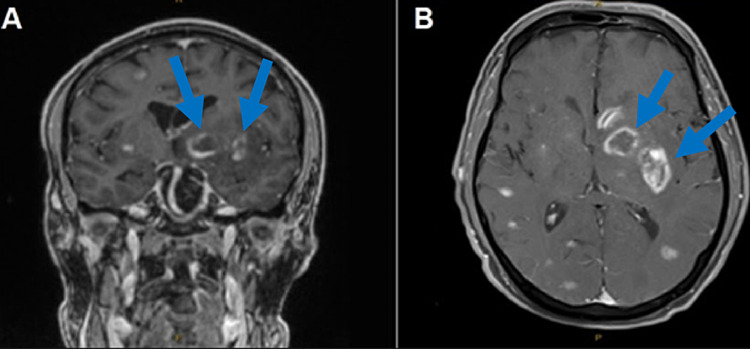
MRI of the brain demonstrating multiple ring-enhancing lesions (blue arrows) in coronal (A) and axial (B) sections.
